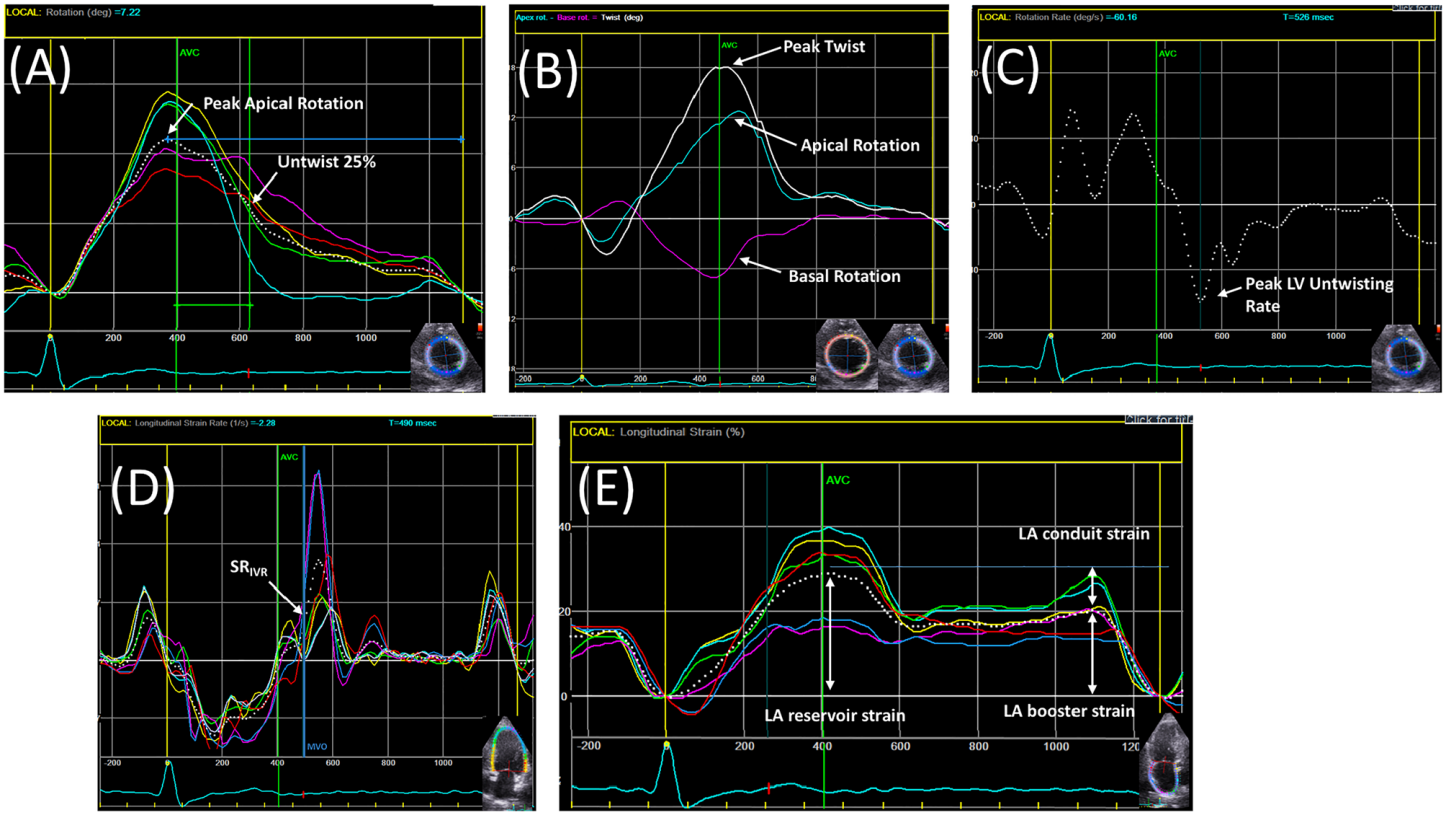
Speckle-tracking measures on echocardiography. a Peak apical rotation and untwist measurements from the apical short-axis view (SHAX). b Basal rotation and apical rotation generates peak left ventricular (LV) twist, measured from the basal-SHAX and apical-SHAX, respectively. c Peak LV untwisting rate, measured from the apical-SHAX. d Peak global longitudinal strain rate during isovolumetric relaxation (SR_IVR_). e Left atrial (LA) strain in the apical 4-chamber view measured as reservoir, conduit, and booster strain. AVC–aortic valve closure; MVO–mitral valve opening
